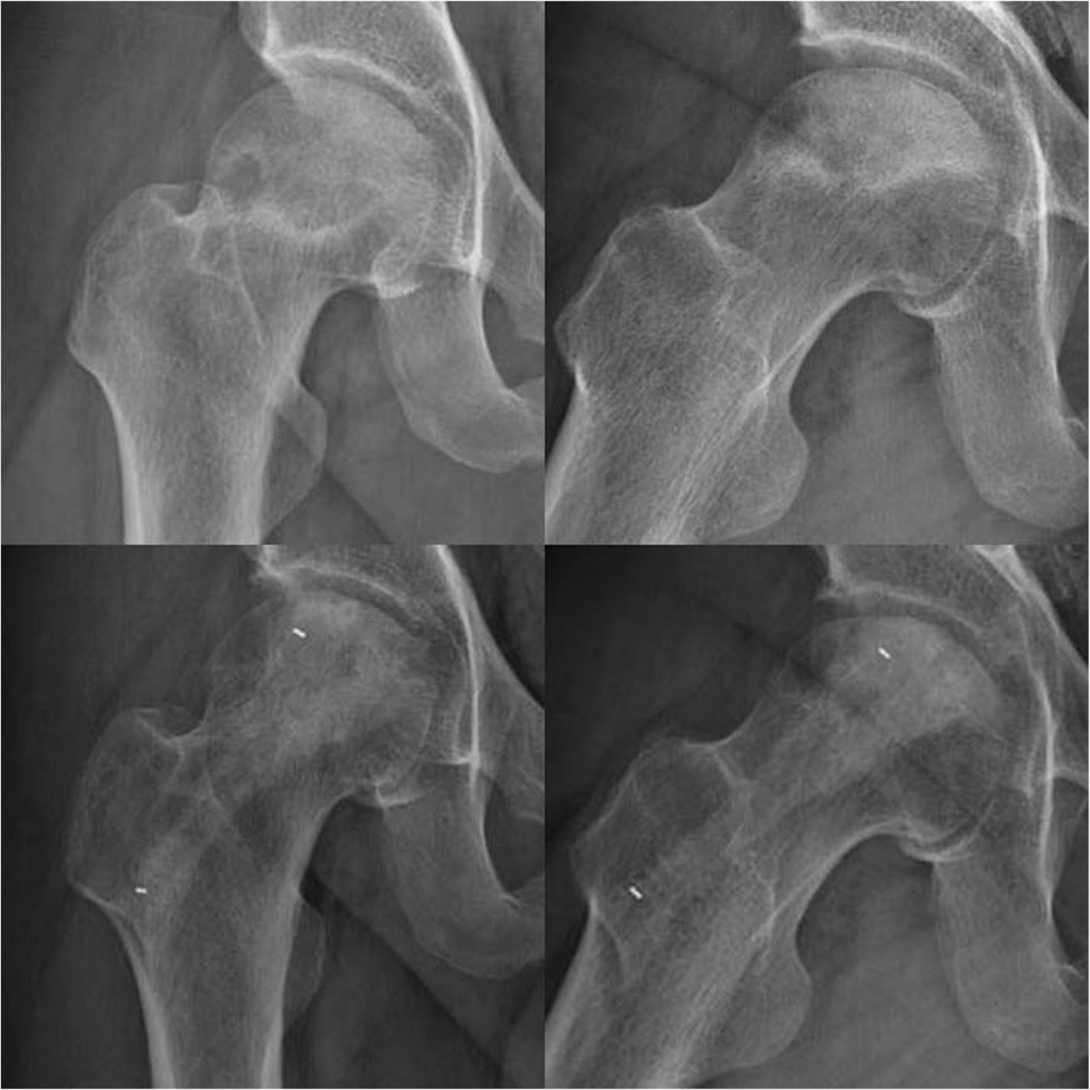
Unilateral radiographs before (top) and at the last follow-up (bottom)
